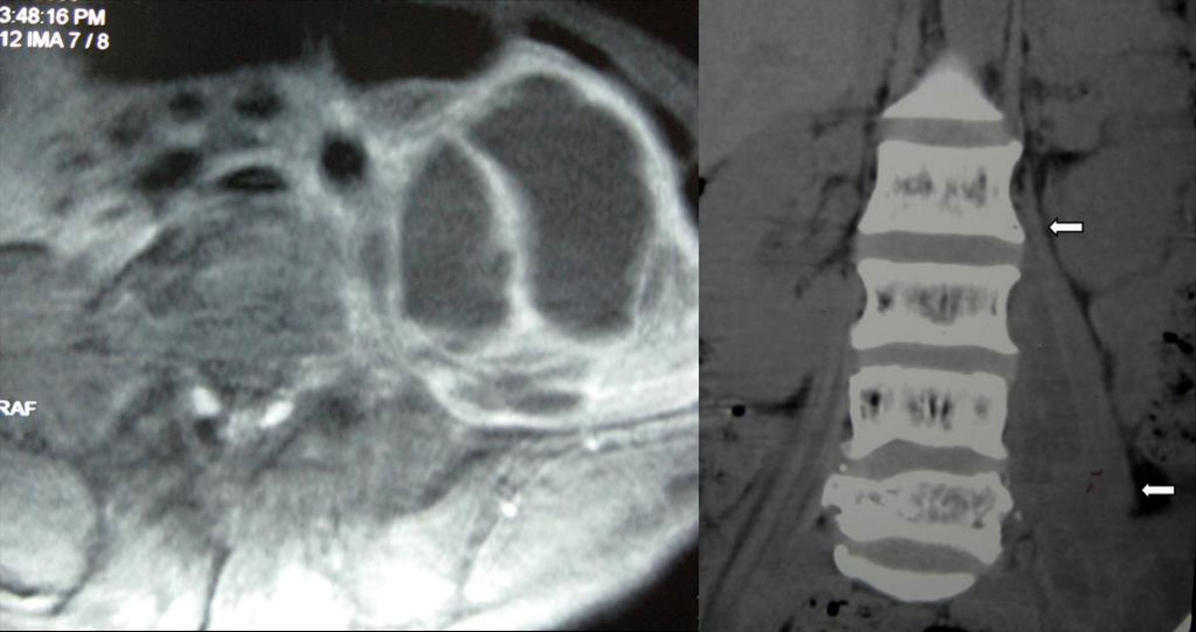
MRI and CT showing the large lesion in the left psoas.
